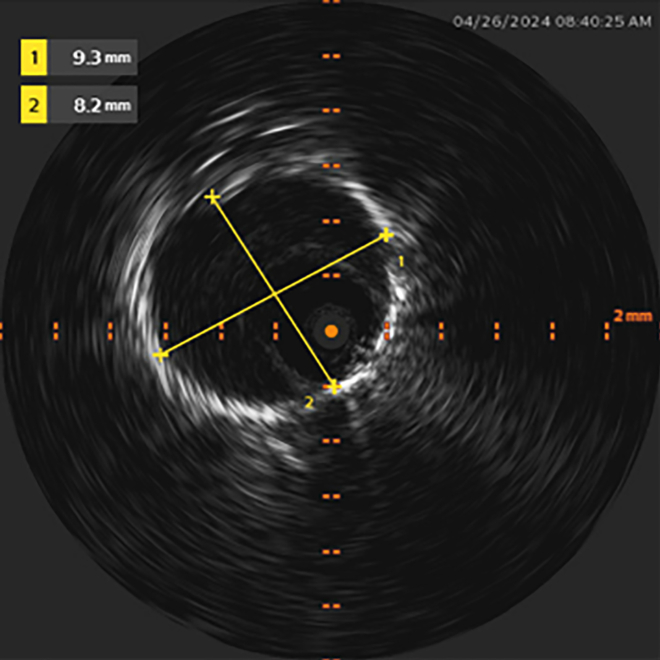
Measurement of LPA Segment Diameter Using IVUS IVUS = intravascular ultrasound; LPA = left pulmonary artery.
